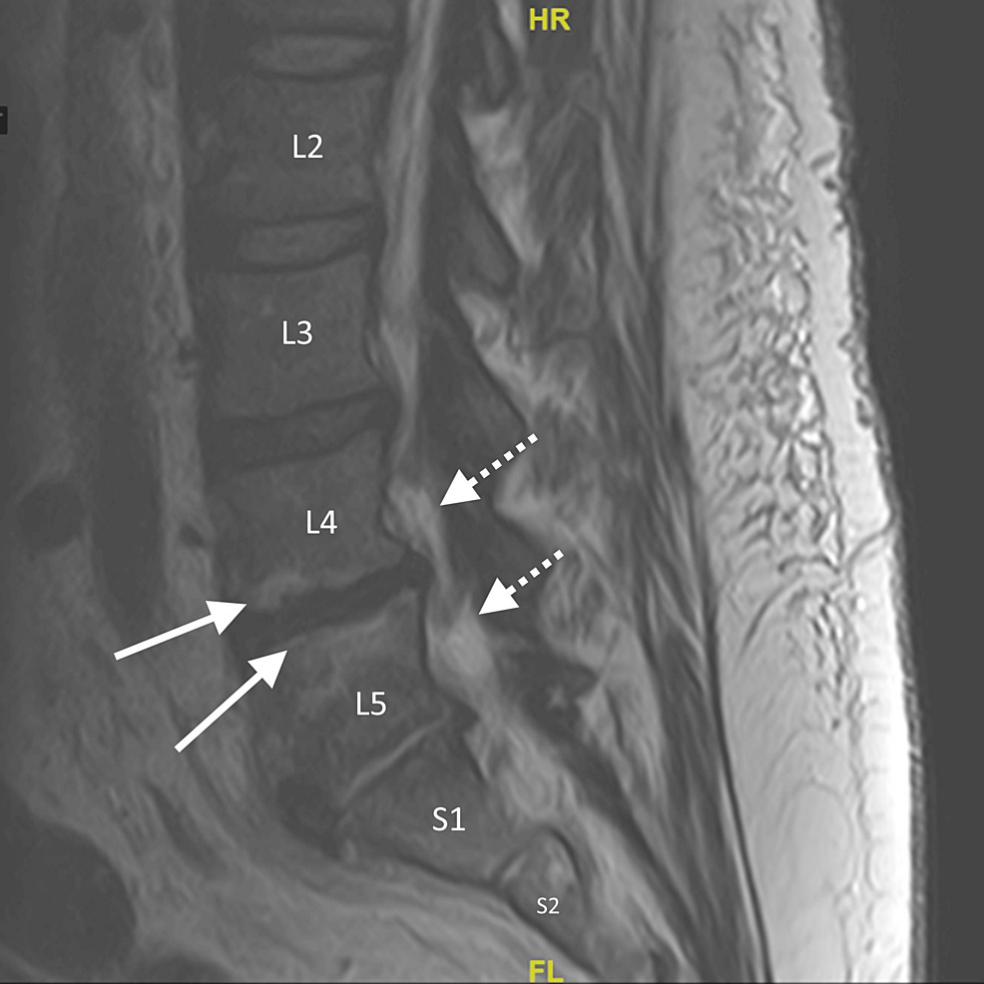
MRI of the patient's lumbar spine with and without contrast showing discitis at L4-L5 (solid arrows) with abnormal enhancement of the anterior paraspinal soft tissues from L4-S2 with enhancement and thickening of the caudal thecal sac and distal roots of the cauda equina likely representing arachnoiditis (dotted arrows)
